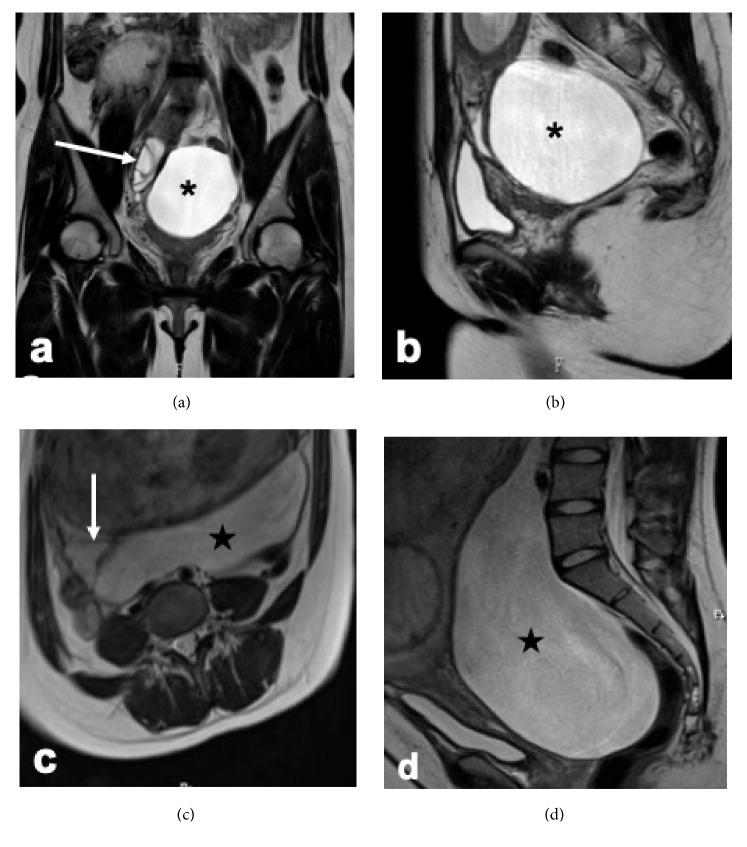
T2-weighed magnetic resonance imaging at 9 (a, b) and 32 (c, d) weeks of gestation. (a) Bilateral pelvic masses with a horizontal view. A right-sided multilocular mass (arrow) and left-sided unilocular mass (asterisk) are shown. We suspected bilateral ovarian tumors at this point. (b) Sagittal view. The left-sided unilocular mass (asterisk) is shown. (c) Horizontal view. The right-sided multilocular mass (arrow) is involved in or at least is located very close to the large mass (star). (d) Sagittal view. The left-sided mass (star) occupies the pelvic cavity.
